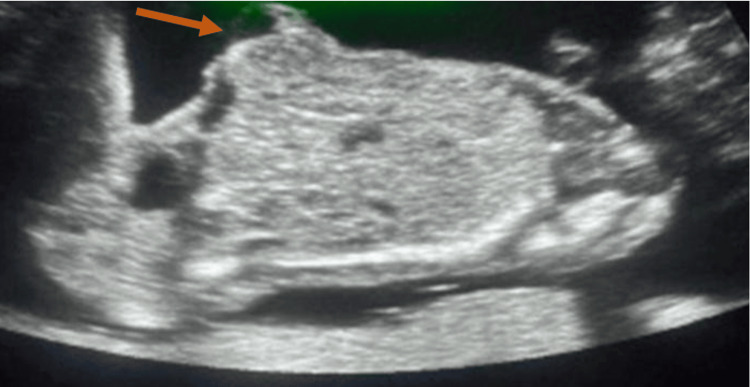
Ultrasound image of the fetal abdomen shows multiple hyperechogenic loops herniating into a membrane covering defect at the level of the umbilicus indicating omphalocele (arrow).
